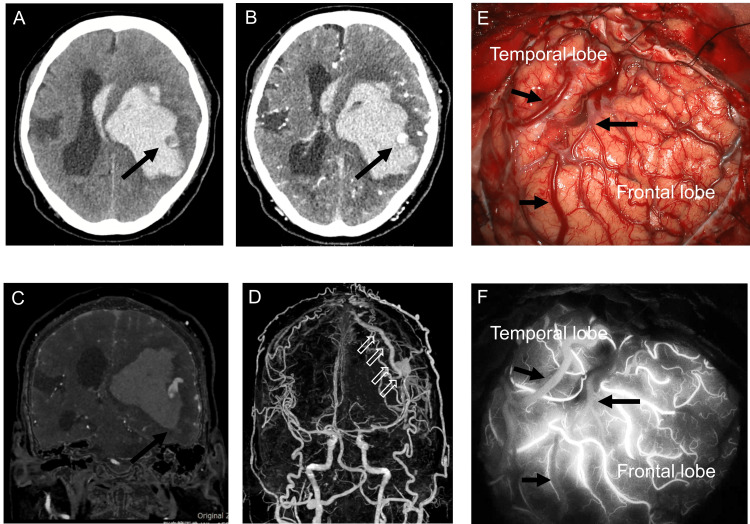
Noncontrast (a) and contrast (b, c) computed tomography revealing left subcortical intracerebral hemorrhage and varix (black arrow). Volume-rendering image (d) showing a dilated cortical vein (open arrow). (e) Intraoperative view during craniotomy. (f) Indocyanine green videoangiography findings. Black arrows indicate the dilated draining vein (“red vein”). The shunt point of the dural arteriovenous fistula (dAVF) was located outside the operative field, making simultaneous hematoma evacuation, decompressive craniotomy, and shunt dissection technically challenging
